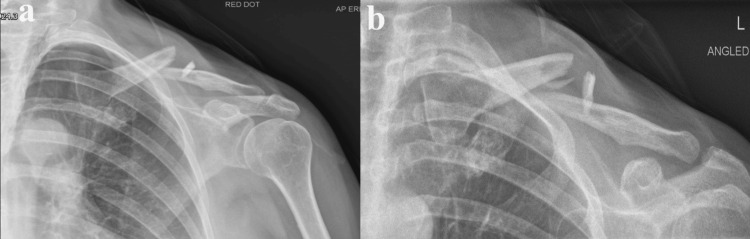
Case 3: Initial left shoulder X-ray exhibiting significant displacement, shortening, and a butterfly fragment a displays the AP view, while b shows the cephalic tilt view. AP: anterior-posterior
